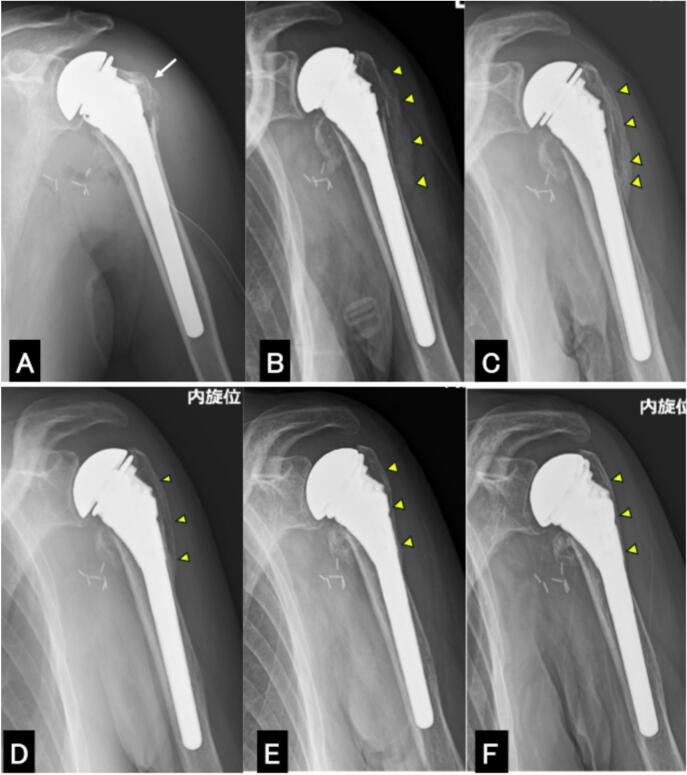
Plain anteroposterior radiographs of the left shoulder: during surgery (A), 16 days after surgery (B), 1 month after surgery (C), 3 months after surgery (D), 6 months after surgery (E), and at one year after surgery (F). A: Anatomical reduction of the greater tuberosity is achieved immediately after surgery (white arrow). B: Vigorous callus formation bridging the greater tuberosity and shaft is shown, confirming bone union (yellow arrowheads). C: The callus size has decreased and consolidation is more evident compared to 16 days after surgery, indicating a mature callus bridge over the greater tuberosity and the shaft (yellow arrowheads). D: A plain radiograph taken three months after surgery shows the beginning of remodelling of the callus (yellow arrowheads). E: A plain radiograph taken six months after surgery shows a reduction in the size of the bony bridge, suggesting progressive remodelling (yellow arrowheads). F: A plain radiograph taken one year after surgery shows no change in the size of the bony bridge, suggesting remodelling was complete by six months postoperatively (yellow arrowheads).
